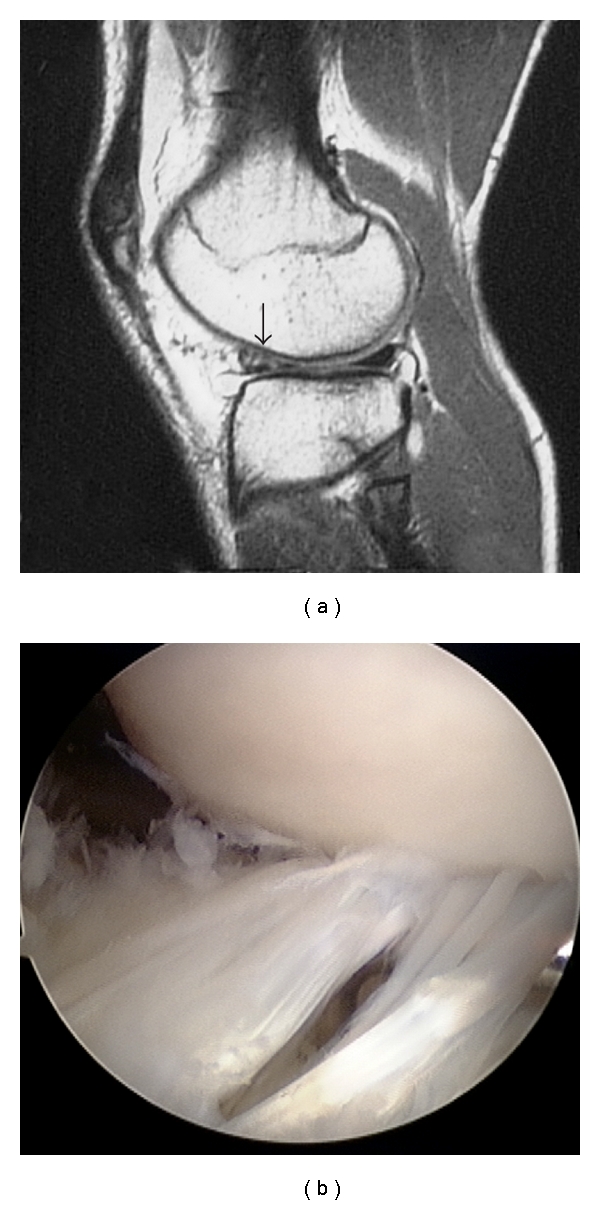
A 17-year-old male senior high school soccer player. (a) Sagittal T2-weighted MRI shows hyperintense signal (arrow) in the anterior horn of the lateral meniscus. (b) Arthroscopic image shows multiple longitudinal tears in the anterior horn of the lateral meniscus.
